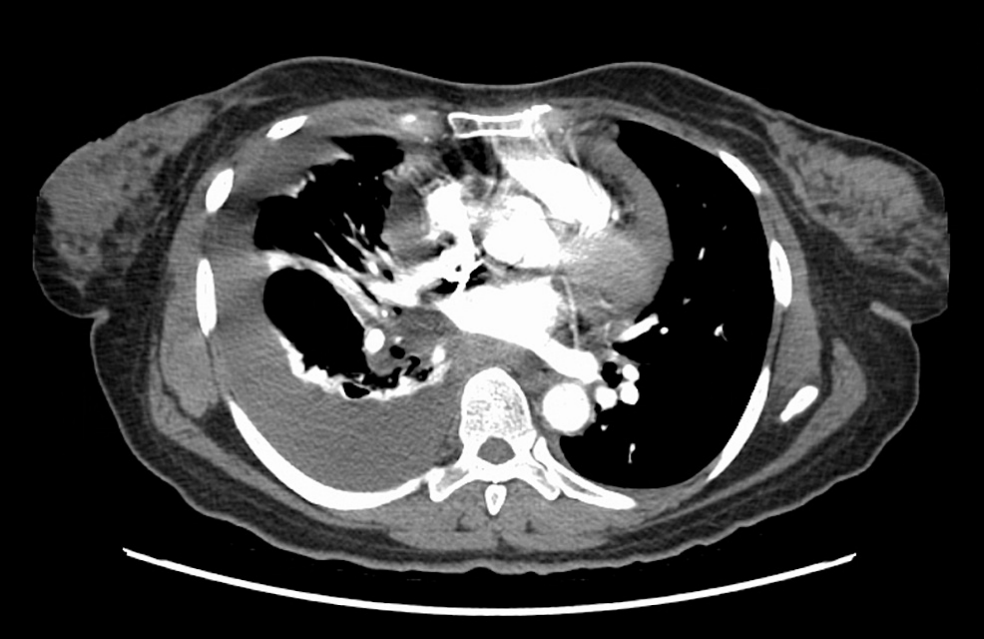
CT angiography of the chest showing pleural and pericardial effusions.
